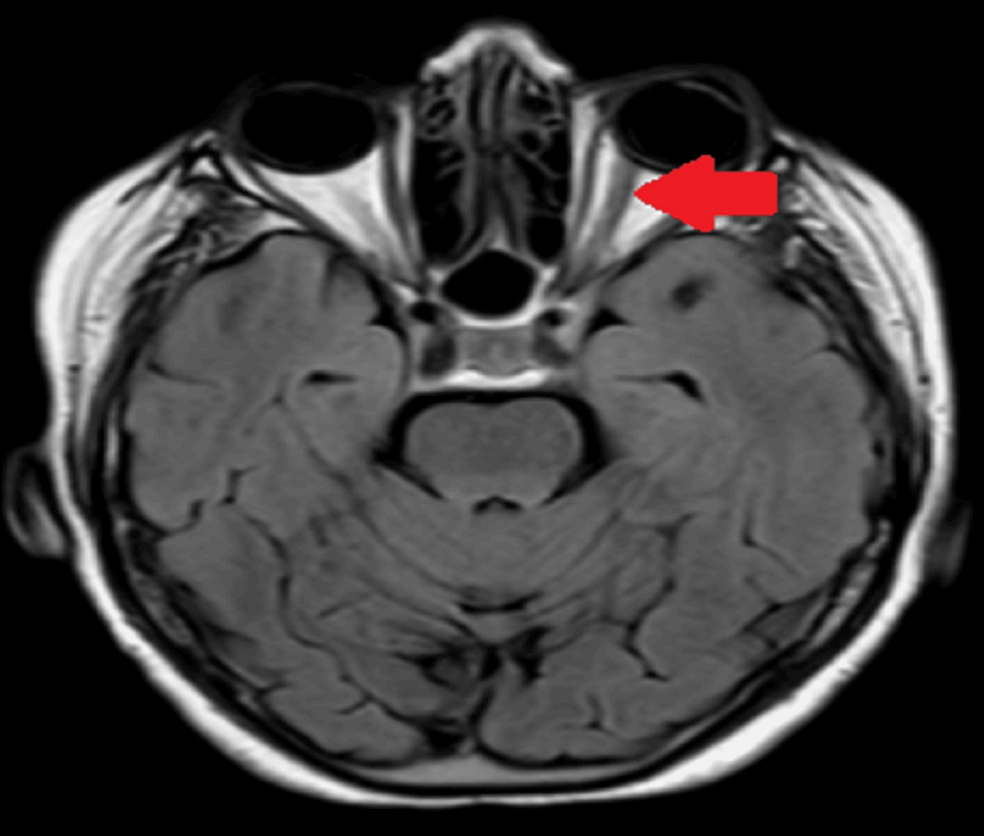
Brain magnetic resonance imaging shows intact brain anatomy and intact optic nerves.
